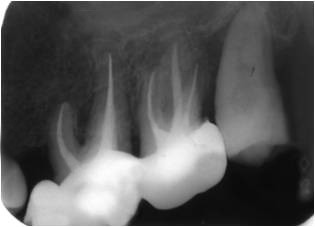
One year follow up radiograph showing resolution of most of the periradicular lesions
